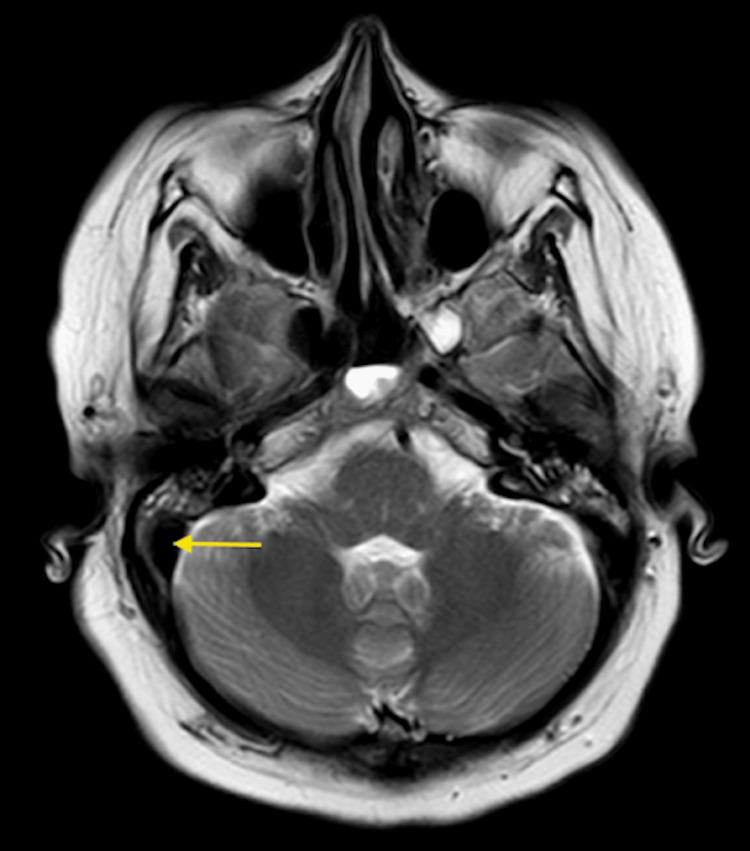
Follow-up MRI Brain with contrast on Day 21 (T2, axial) demonstrates signal void at site of previous thrombus, with no features of ongoing DVST. DVST: dural venous sinus thrombosis
